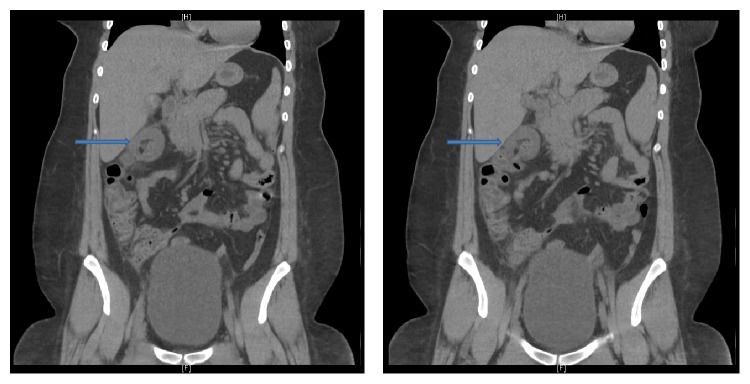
CT abdomen demonstrating colocolic intussusception at the hepatic flexure (arrow).
